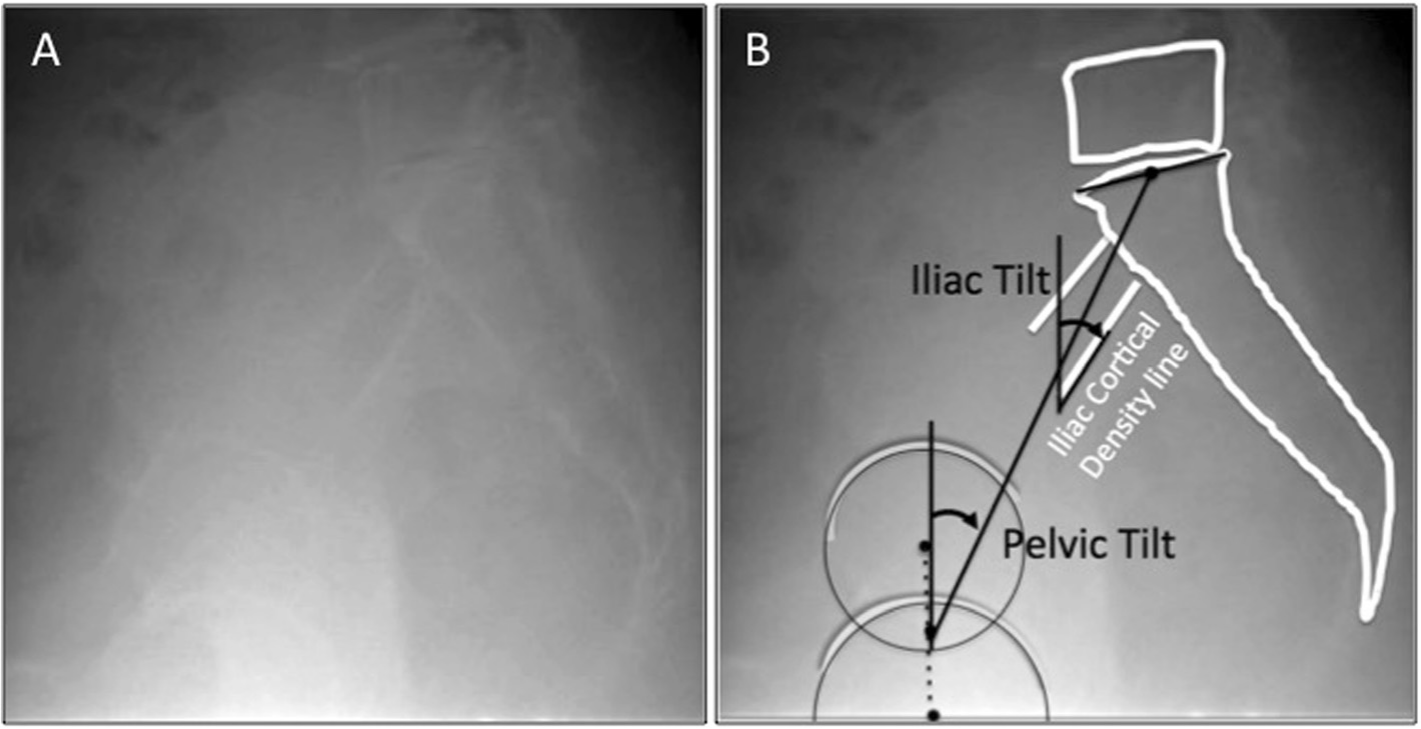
Iliac cortical density line and the iliac tilt. In the lateral X-ray, the iliac cortical density line can be seen as a *straight line* several centimeters in length around the anterior border of S1, which is considered to reflect the thickness of the cortical bone of the ilium along with the arcuate line of the ilium (a). The iliac tilt (IT) was defined as the *angle* between the lower (the farther side to the film) iliac cortical density line and the vertical (b)
